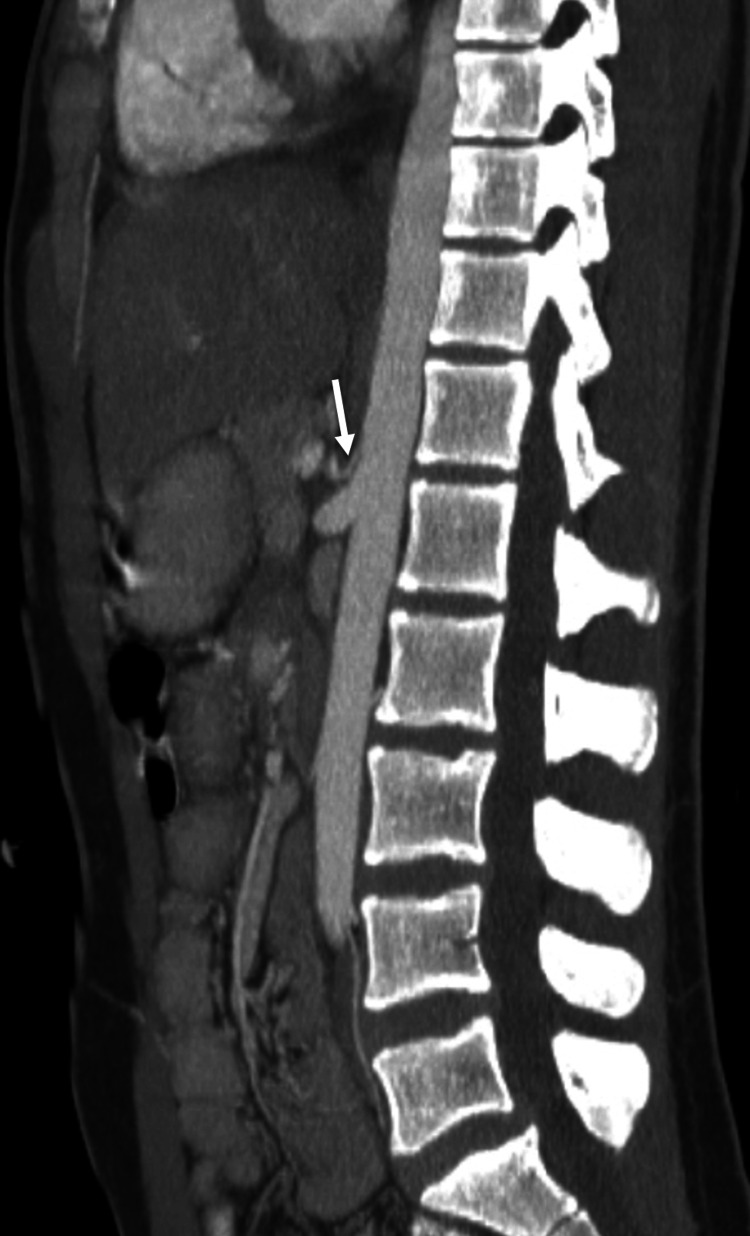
Sagittal MIP CT image highlighting the anteroposterior orientation and attenuated celiac trunk, demonstrating its limited contribution to visceral arterial supply.
